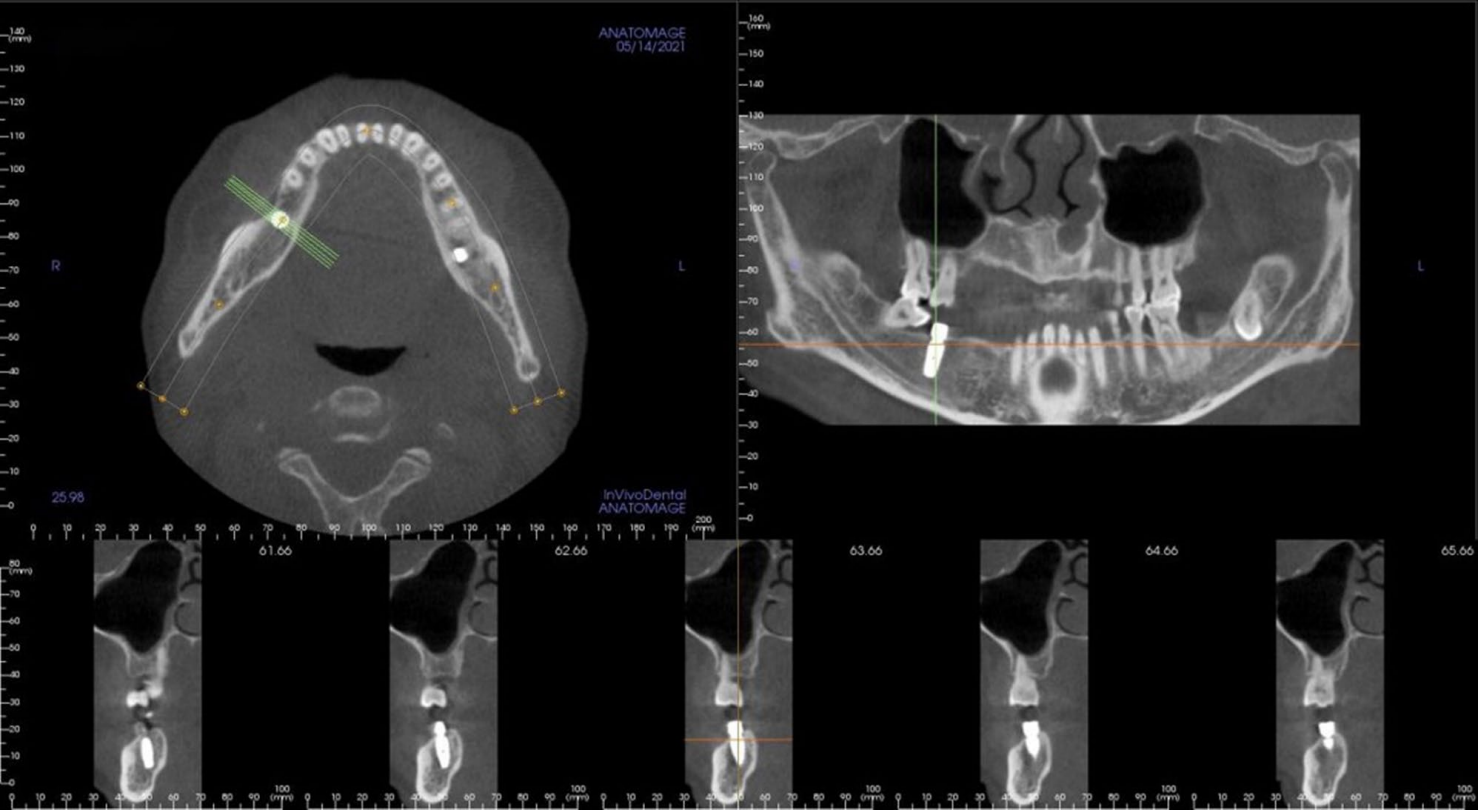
CBCT of dynamic navigation assisted immediate implantation of the mandibular posterior teeth region
